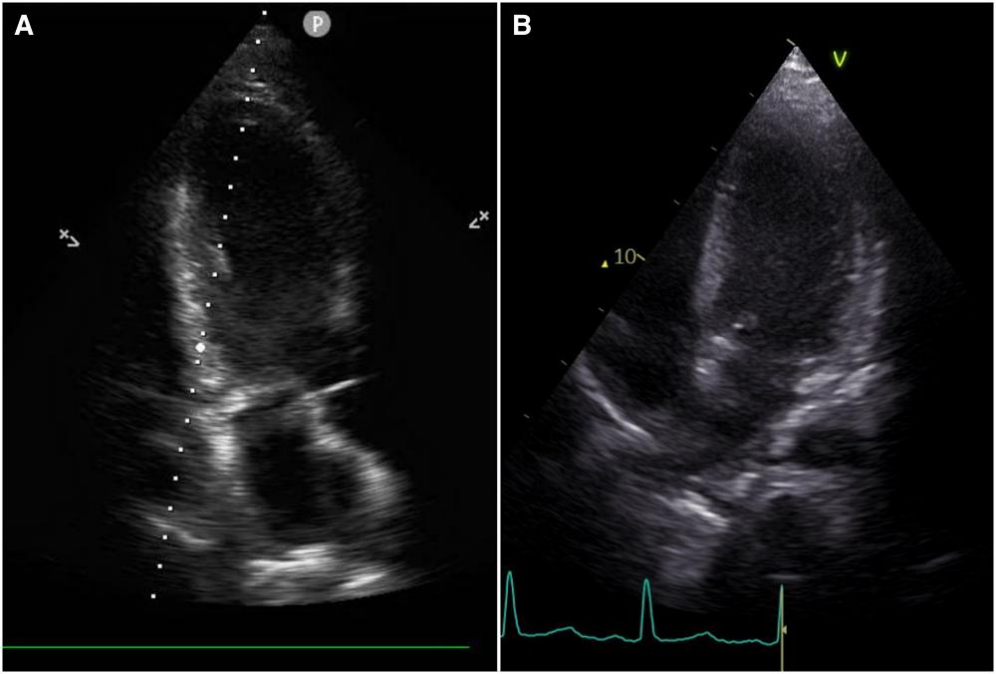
Echocardiography demonstrating hyperechogenicity of the myocardium (*A*), compared with normal myocardial echogenicity on an earlier examination (*B*).
